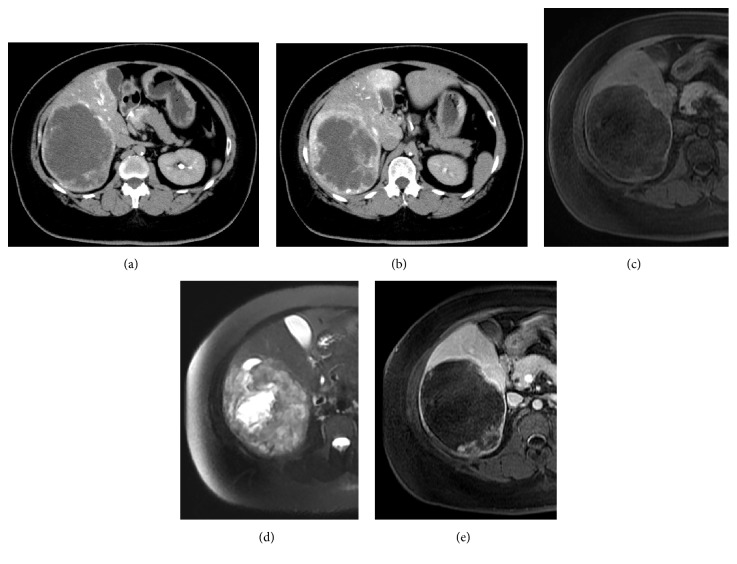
Abdominal computed tomography during hepatic angiography and magnetic resonance imaging. Arterial phase CT scan shows a geographic lesion in the right lobe of the liver with a rim and nodular enhancement (a), and the delayed phase of CT reveals heterogeneous enhancement in the peripheral area of the mass with a gradual centripetal enhancement pattern (b). The tumor shows low signal intensity on T1-weighted images (c) and some high-signal intensity nodules on T2-weighted images (d). EOB-MRI shows no uptake in the corresponding area (e).
